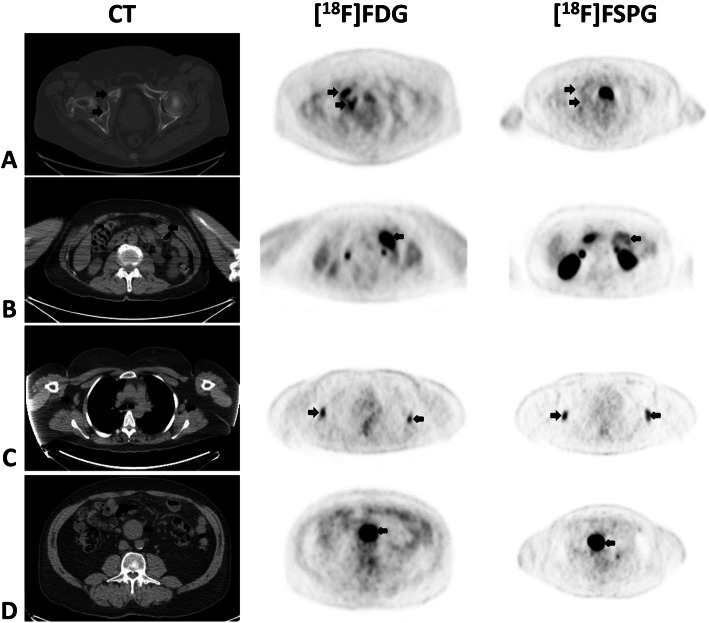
Combined image set shows four representative subjects with non-Hodgkin lymphoma (NHL). Displayed from left to right are the axial CT (soft-tissue window shown at various cranio-caudal levels), [^18^F]FDG PET, and [^18^F]FSPG PET images. The first subject (row a) with diffuse large B cell lymphoma has a large soft-tissue mass in the right hip invading the bone, the second subject (row b) with follicular lymphoma has a large mesenteric lymph node on the left, the third subject (row c) with mantle cell lymphoma has small bilateral axillary lymph nodes, and subject 4 (row d) with mantle cell lymphoma has a large central mesenteric mass. The black arrows indicate the lesions. Any unmarked uptake on the PET scans is physiologic. The lesions are seen on all modalities, although the [^18^F]FSPG PET scan shows very little uptake for some of the lesions (SUV right hip mass (a) 11.3 and 2.9, left mesenteric lymph node (b) 9.2 and 3.2, right axillary lymph node (c) 3.5 and 1.1, and central mesenteric mass (d) 11.1 and 21.9, for [^18^F]FDG and [^18^F]FSPG, respectively)
